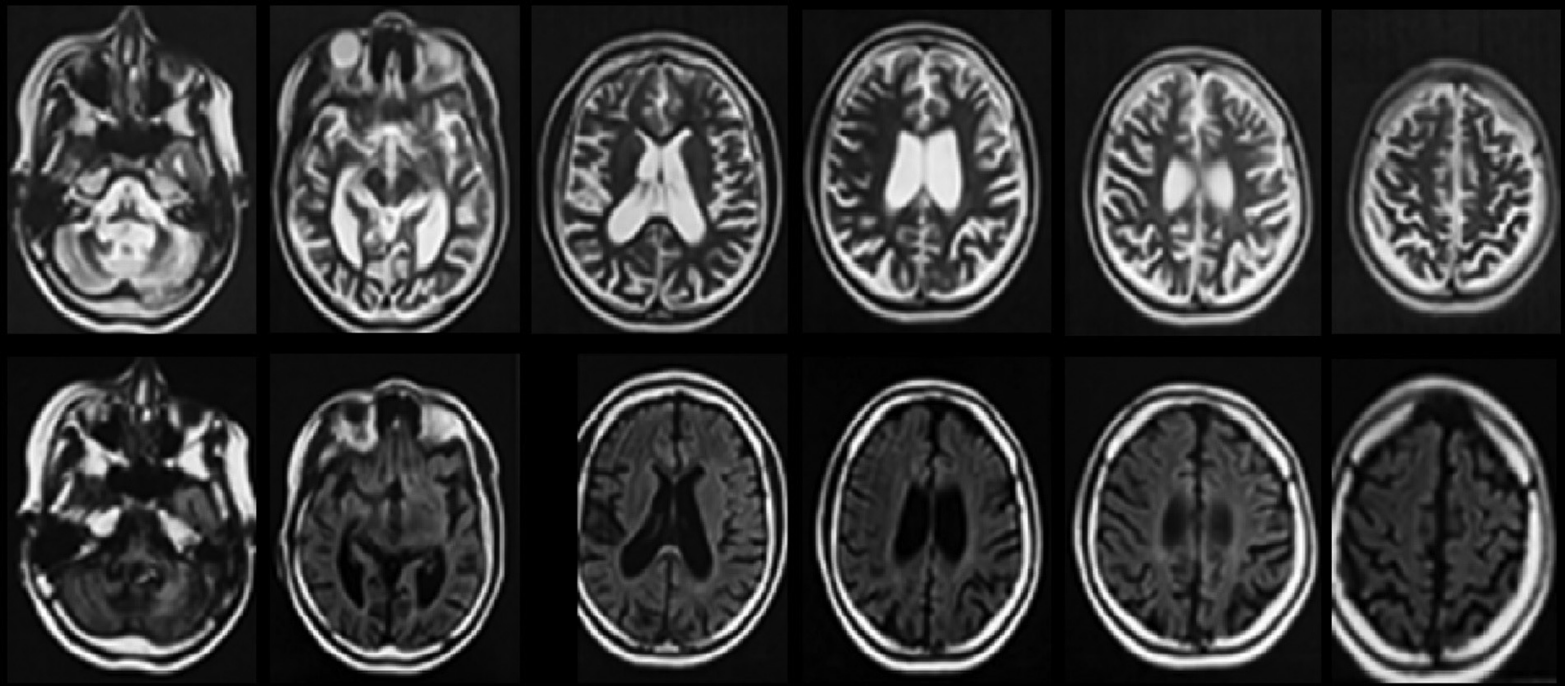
Brain MRI in a patient with CLN2 disease. Axial T2-weighted (first line) and FLAIR (second line) slices show cortical-subcortical atrophy with ex-vacuum ventricular dilatation and cerebellar atrophy.
